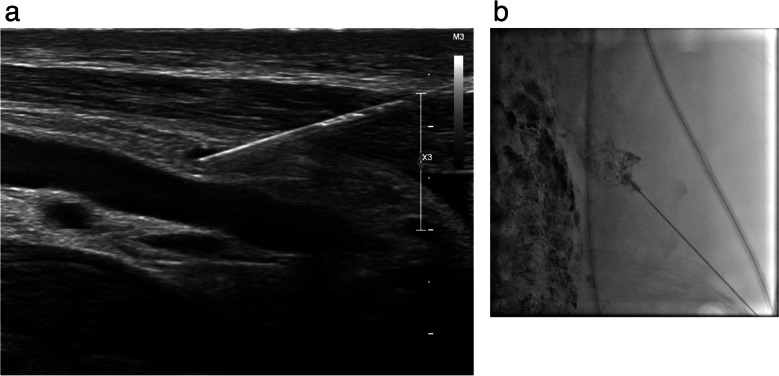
a, b Ultrasound guided puncture of a mesenteric lymph node (a) and mesenteric lymphangiography (b)
